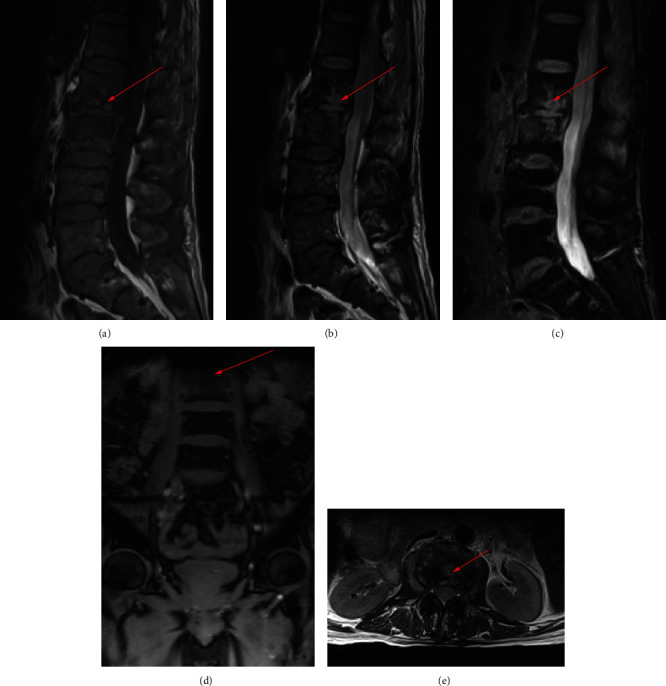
MRI examination of a patient with spinal tuberculosis. The arrow points to the location of the lesion. (a) MRI image in the sagittal T1 sequence. (b) MRI image in the sagittal T2 sequence. (c) MRI image of T2 lipid compression sequence in sagittal position. (d) MRI image in coronal position. (e) MRI image in cross-section.
